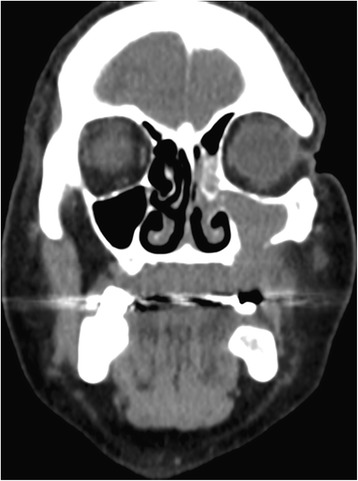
Staging CT scan confirming maxillary destruction but preservation of the orbital floor
